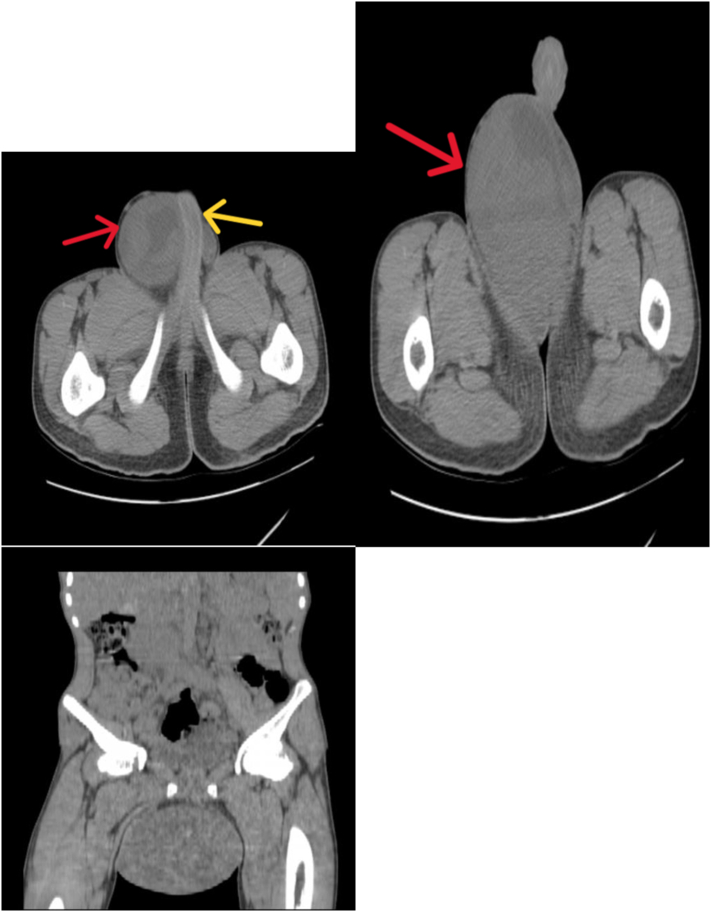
Pre-contrast CT scan images depicting a huge right scrotal mass (red arrows) pushing the penile shaft (yellow arrow) and extending distally between the thighs.
